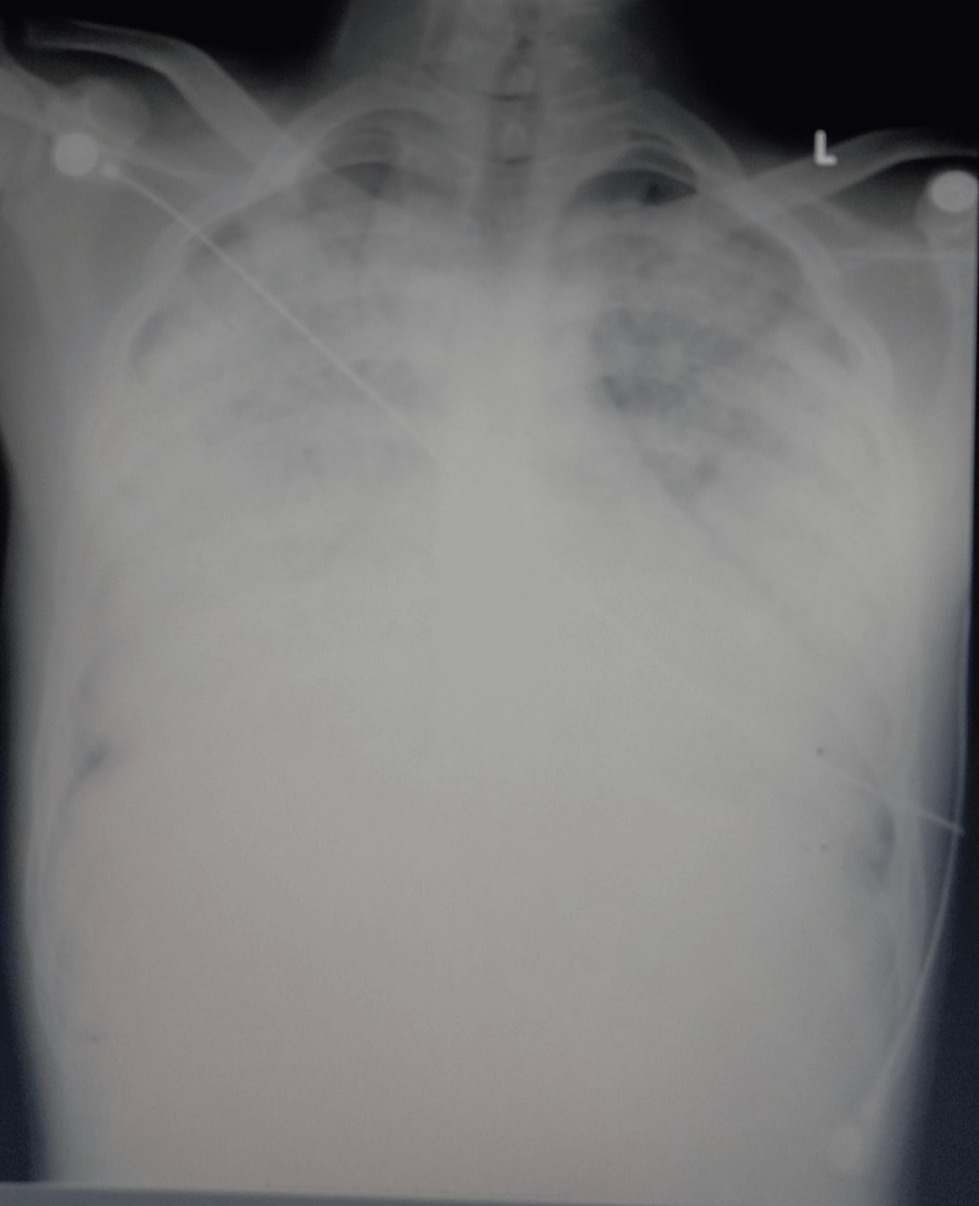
Chest X-ray showing bilateral infiltrates.
